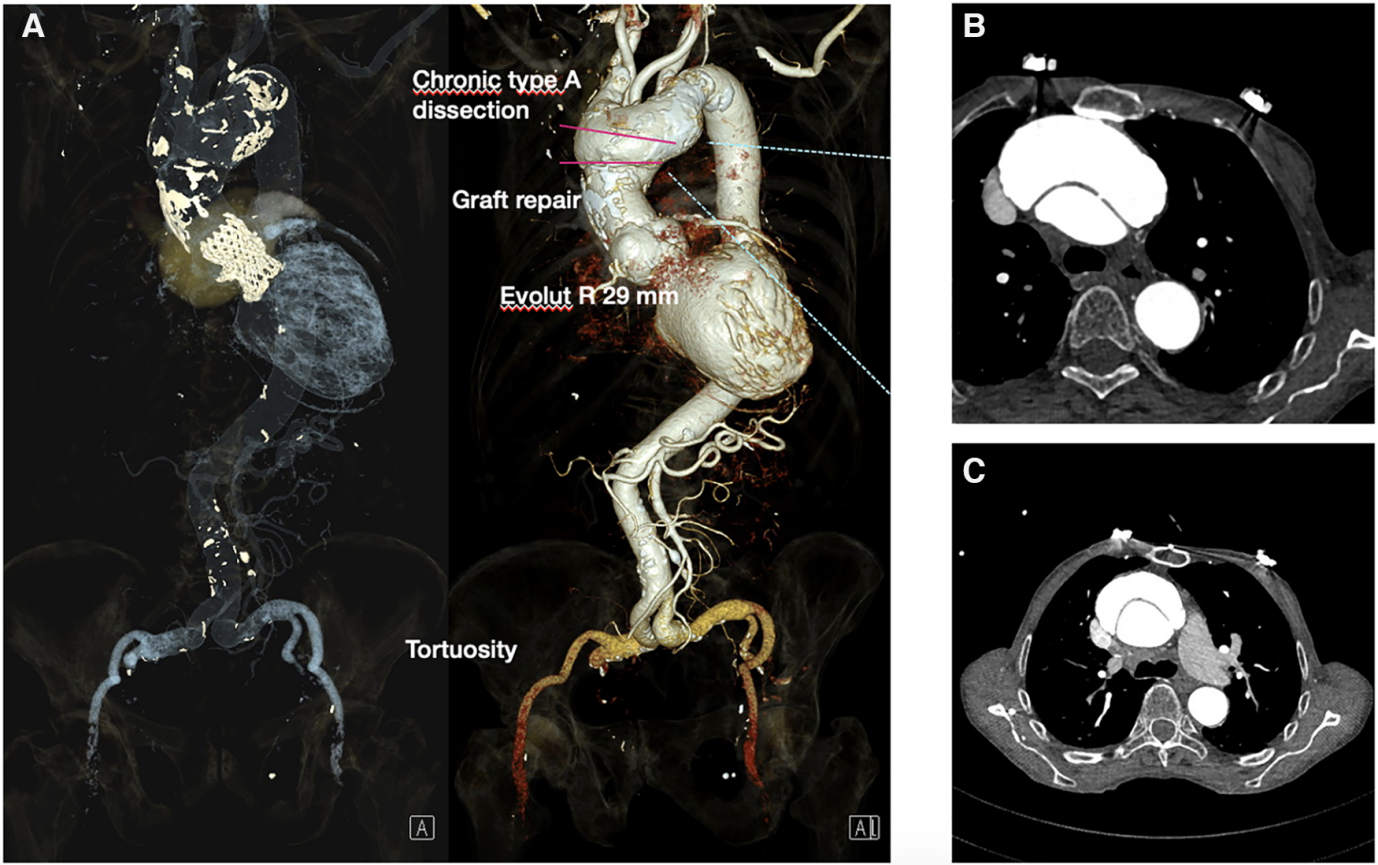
CT angiography following TAVI protocol: (A) EvolutR TAVI valve with graft repair and aortic aneurysm with type A dissection of the ascending aorta and proximal arch. Severe tortousity of the iliac arteries. (B,C) Type A aortic dissection in the transverse plane.
